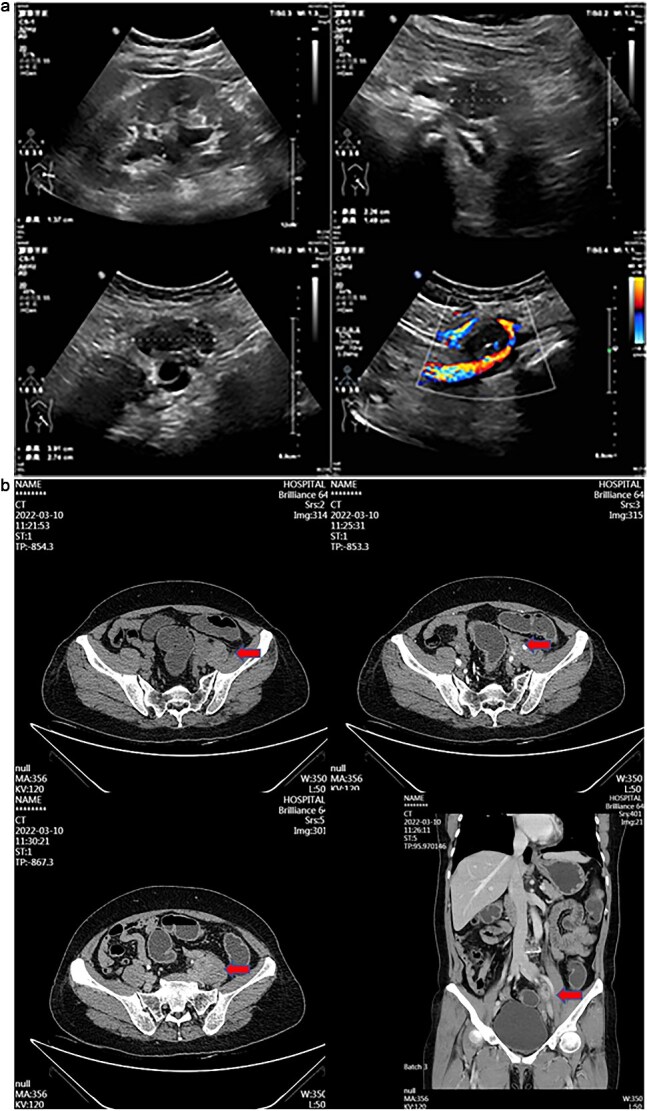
(a) Transabdominal ultrasound image showing hypoechoic and mixed-echoic masses adjacent to left iliac vessels with associated mild left Hydronephrosis and mid-ureteral compression. (b) Contrast-enhanced CT image demonstrating ring-enhancing left adnexal mass and left iliac vessel nodule with associated ureteral compression and mild hydroureteronephrosis.
